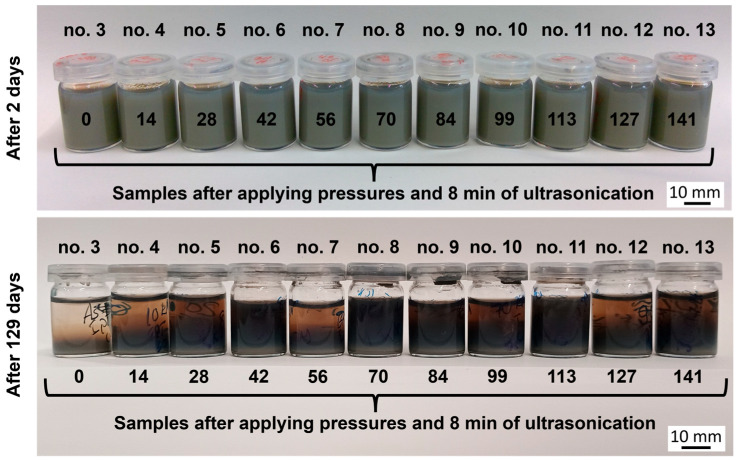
Boron carbide particles sedimented in deionized water after being left for 2 days and 129 days at room temperature, with different pressures and 8 min of ultrasonication subsequently applied.
